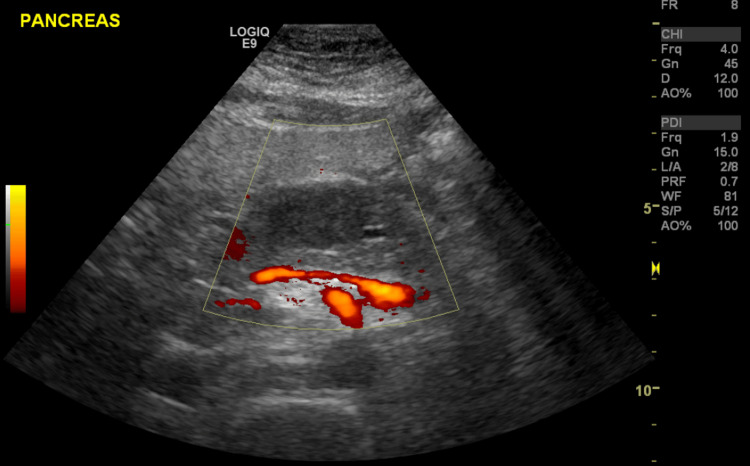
Ultrasound-guided biopsy of the pancreatic mass
